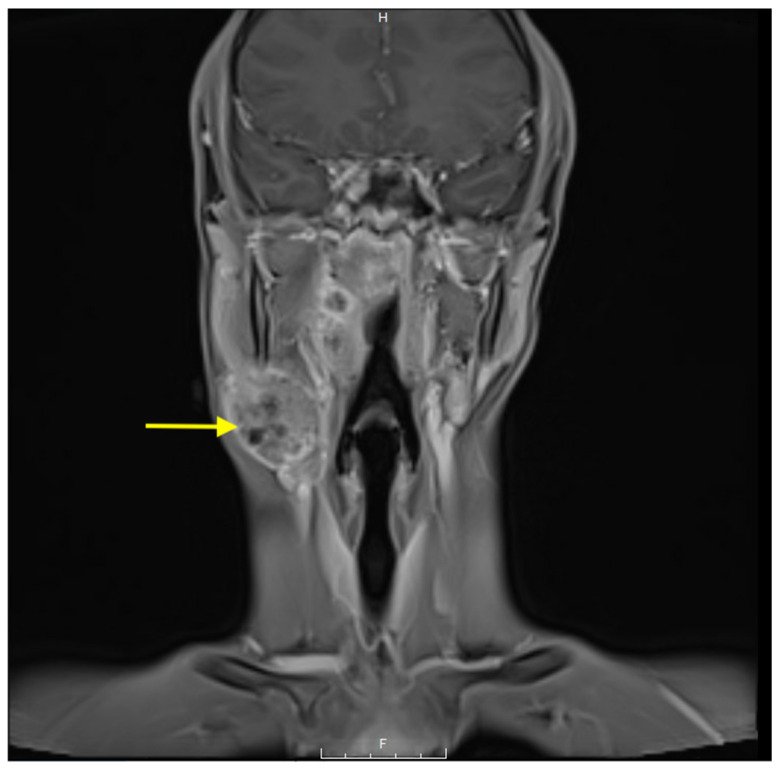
Coronal T1-weighted post-contrast MRI demonstrating a large, enhancing mass (indicated by the arrow) in the right nasopharynx, extending into the parapharyngeal space, indicative of nasopharyngeal carcinoma.
